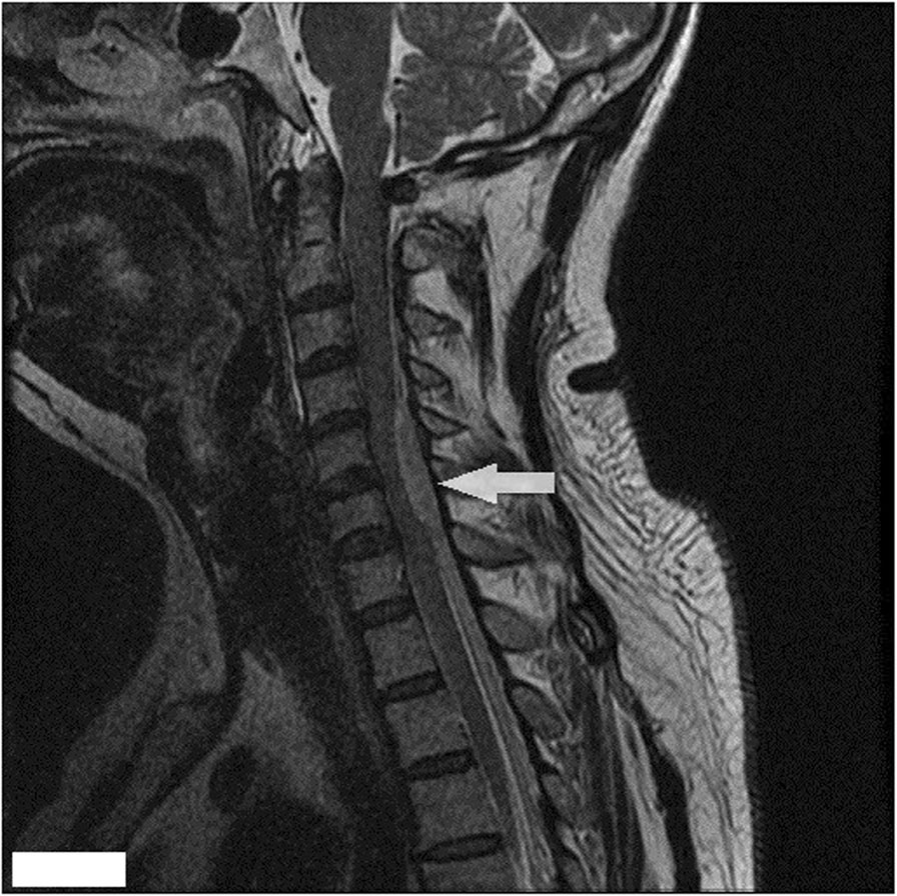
Sagittal MRI of the cervical spine (T2-weighted image). An area of increased intensity from the C3–C7 levels can be seen
